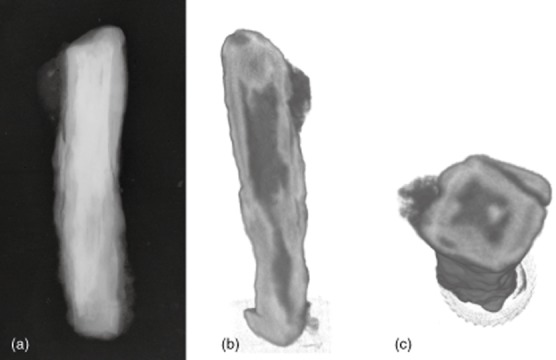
An X-radiograph of CPF08_062 (a), and a longitudinal cross-section (b) and a transverse cross-section (c) through the neutron tomography image of CPF08_062. Darker grey values represent higher neutron attenuation; that is, larger atomic density of Fe. This is due to the different densities of metallic iron and corrosion products.
